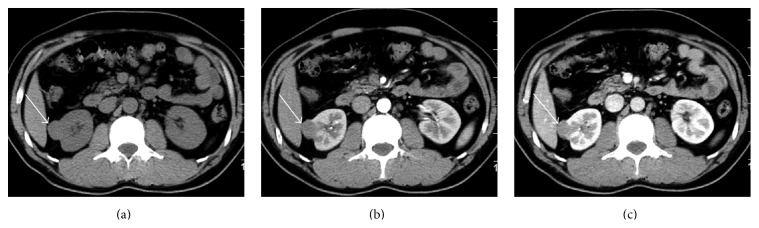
Type 1 papillary renal cell carcinoma in a 39-year-old asymptomatic man. (a) Abdominal plain CT scan shows a round well-defined isodense (40 HU) nodule of 2.5 cm in size in the cortex of right kidney. (b) The tumor is enhanced slightly and homogeneously (to around 58 HU) during corticomedullary phase without necrotic or cystic areas in the lesion. (c) The tumor continues to be enhanced homogeneously (to around 78 HU) during the nephrographic phase.
